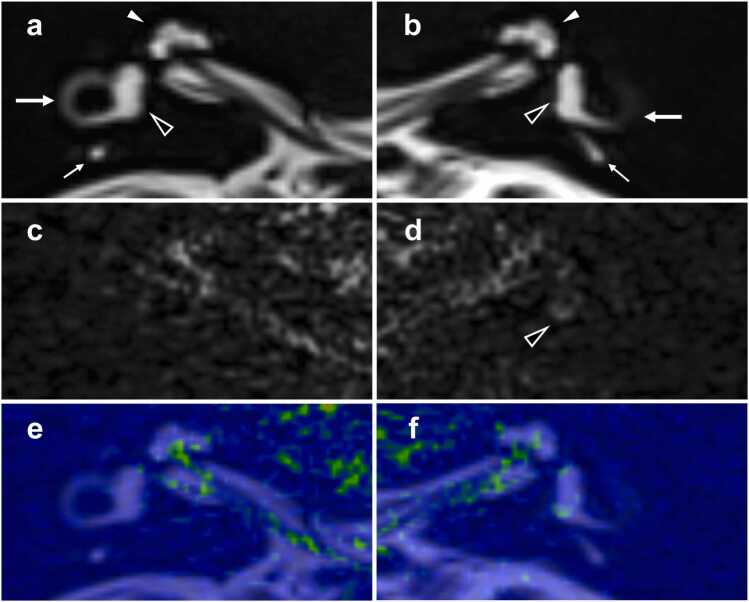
32-year-old male with ipsilateral inner ear high signal on non-contrast 3D FLAIR imaging. a-b MRC: Hyperintensity is observed within lymph fluid located in the cochlea (white arrowhead), vestibule (black arrowhead), posterior semicircular canal (small arrow), and lateral semicircular canal (arrow) on the right (a) and left (b) sides. c-d Non-contrast HT2-FLAIR imaging: Abnormal high signal is depicted in the left vestibule (black arrowhead); this signal is higher than that of the CSF in the cerebellopontine angle cistern without sharply delineated borders (Grade 1). In contrast, the right inner ear shows low signal intensity, similar to the CSF in the cerebellopontine angle cistern, representing a normal signal (Grade 0). e-f Fusion images generated from MRC and non-contrast HT2-FLAIR image: The MRC image provides anatomical reference in grayscale, while the non-contrast HT2-FLAIR image is superimposed using a colormap to highlight regions of increased signal intensity. Abbreviations: 3D *three-dimensional*, FLAIR *fluid attenuated inversion recovery*, MRC *MR cisternography*, HT2-FLAIR *heavily T2‑weighted 3D FLAIR*, CSF *cerebrospinal fluid*.
